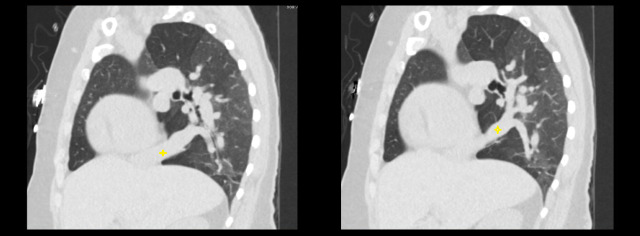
Computed tomography scan of chest (sagittal reconstruction) demonstrating the Scimitar vein (marked by the yellow cross).
